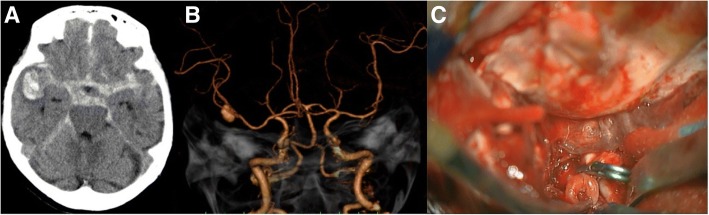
SAH due to right middle cerebral artery aneurysm. Preoperative CT and 3D- CTA and surgical view shows the details of SAH. a Preoperative head CT showing diffuse subarachnoid hemorrhage with right temporal hematoma. b Preoperative 3D-CTA revealing a right middle cerebral artery aneurysm, which is 12 mm in a diameter. c Ruptured right MCA aneurysm was clipped with Sugita titanium clip (arrow). CT: computed tomography, CTA: CT angiography
